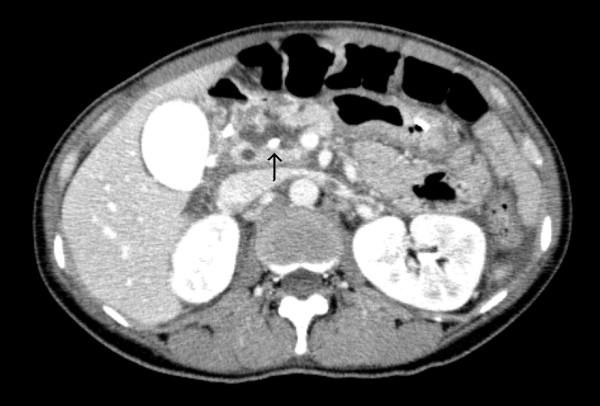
A computed tomography scan after endoscopic retrograde cholangiopancreatography revealed endoscopic nasopancreatic drainage tube (arrow) placed into pancreatic duct, and there was no exacerbation of pancreatic injury or fluid collection.
